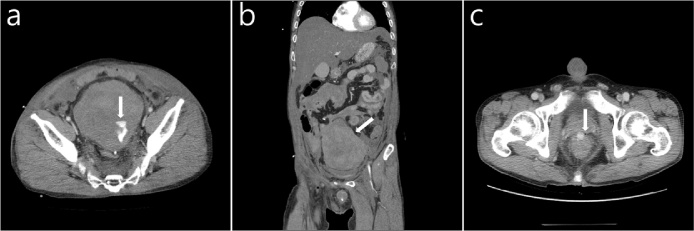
(a) Initial contrast-enhanced axial computed tomography image showing the perirectal hematoma with extravasated blood (arrow); (b) Initial contrast-enhanced coronal image showing the perirectal hematoma with hemoperitoneum; (c) Initial contrast-enhanced axial computed tomography image showing the pseudoaneurysm (arrow).
